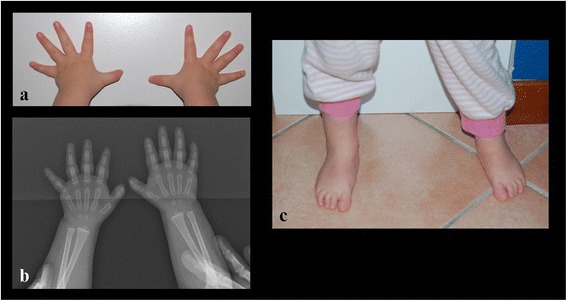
Hands showing tapering fingers and mild bilateral hypoplasia of the distal phalanges of the first and second finger (part a), confirmed by X-ray (part b); small feet with brachydactyly (part c).
